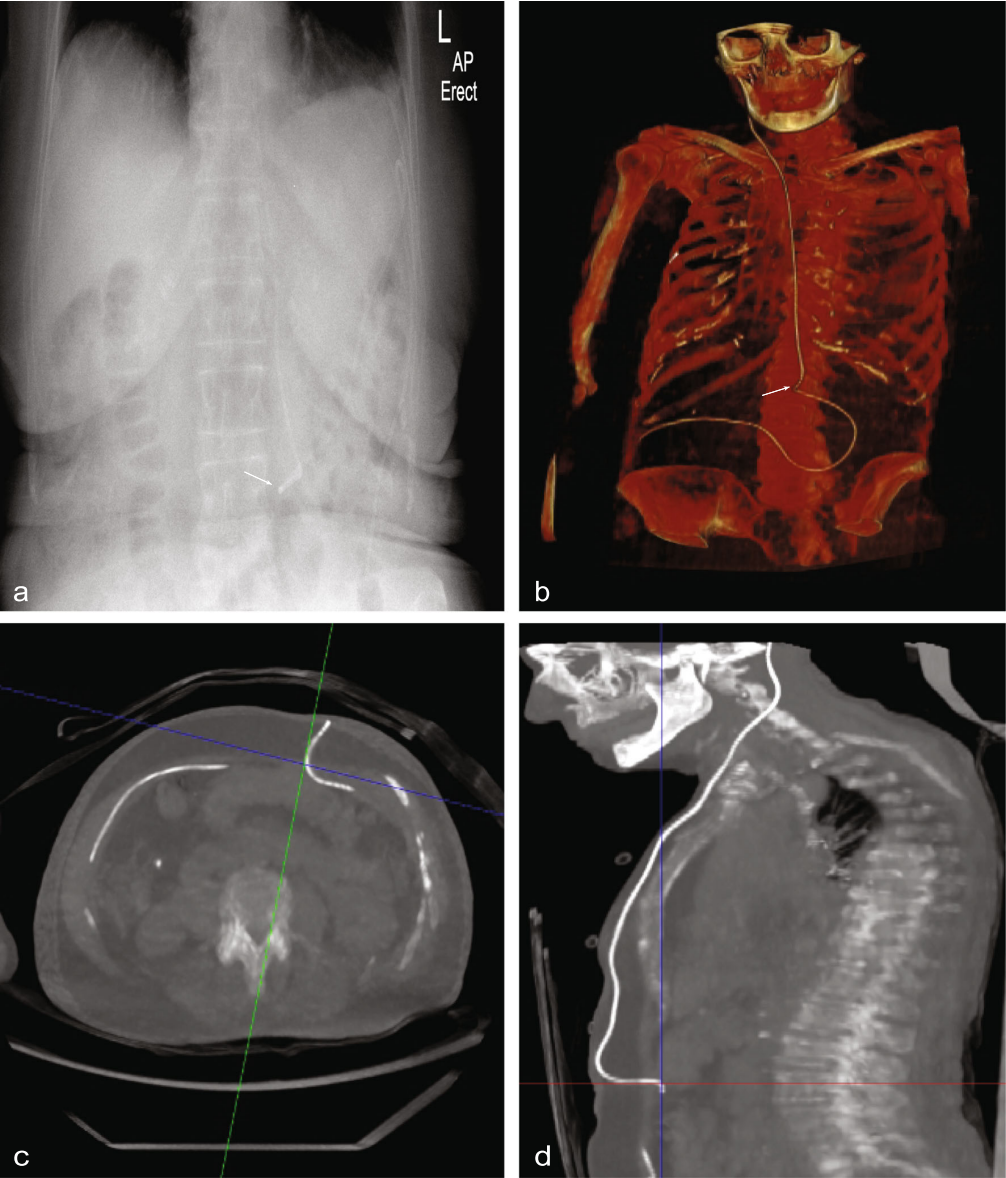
Specificity of ULD CT with pure IR for shunt evaluation. Abdominal radiograph portion of a plain radiographic shunt series (PRSS) demonstrating a kink in the shunt tube (*arrow* in a). ULD CT with pure IR three-dimensional reconstruction confirming the presence of a contour change in the shunt tubing at the corresponding level (*arrow* in b). Axial (42-mm reconstructed slice thickness) and coronal (34-mm reconstructed slice thickness) ULD CT images showing that the ‘kink’ seen on plain radiograph correlates with the peritoneal entry site of the shunt and was therefore a false positive (c and d). *CT* Computed tomography, *IR* Iterative reconstruction, *PRSS* Plain radiographic shunt series, *ULD* Ultralow dose
